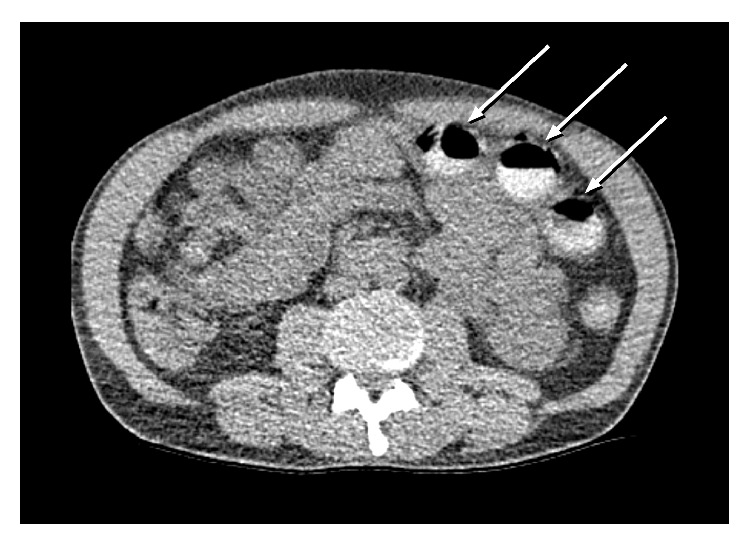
CT scan showing three of four, round, foreign bodies (arrows) in the left transverse colon.
